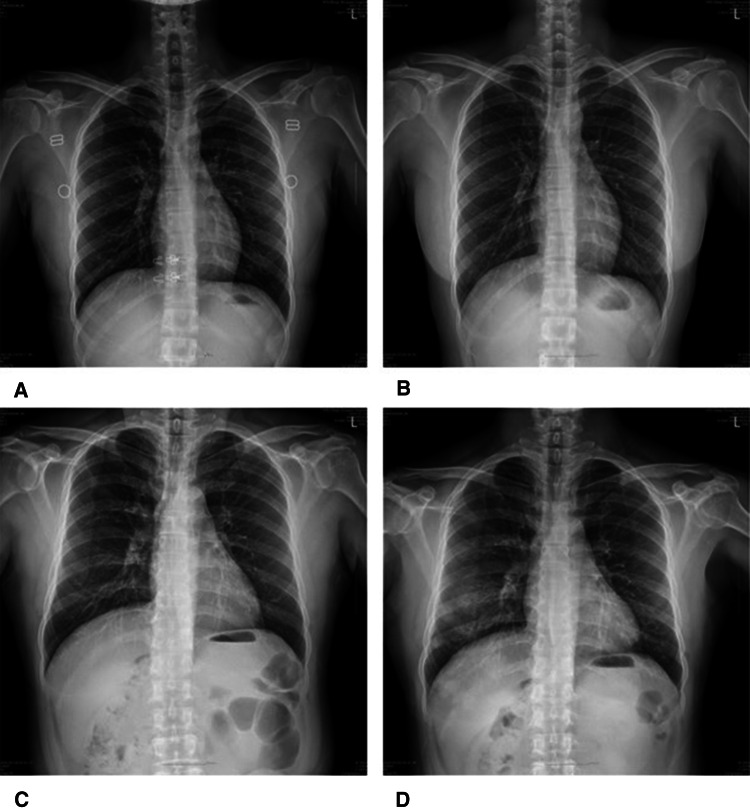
Serial chest radiographic findings of COVID-19 patients. A A 28-year-old woman without any symptoms. Normal finding of chest radiograph. B Same patient as in image (A), taken 4 days after, shows normal finding of chest radiograph. C A 59-year-old man with symptoms of COVID-19 during hospitalization. Normal finding of chest radiograph. D Same patient as in image (C), taken 7 days after, shows newly developed consolidation and GGO at both lower and right mid-lung field
